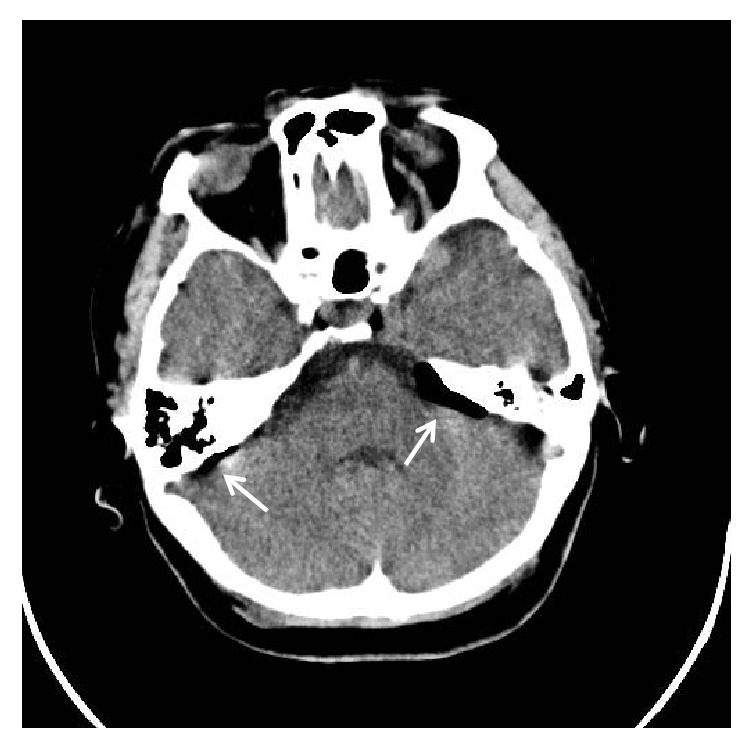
Axial CT brain demonstrating pneumocephalus in the posterior cranial fossa (arrowhead).
